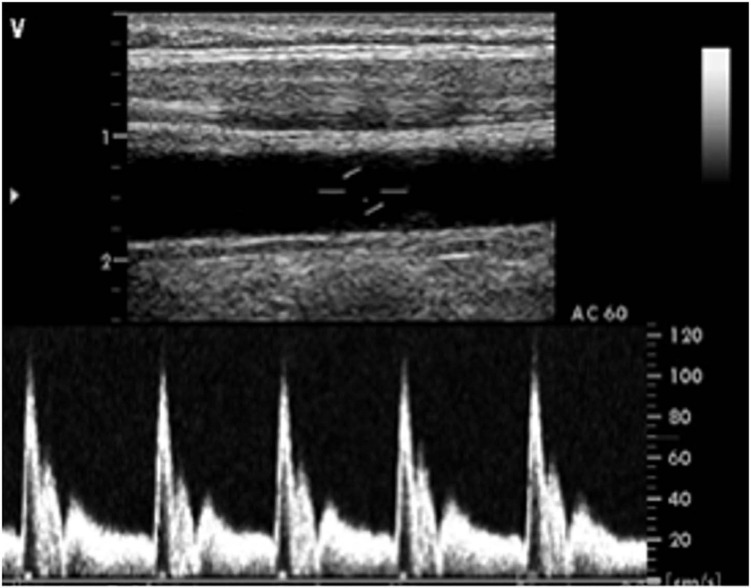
Vascular duplex sonography scan of the left carotid artery showing the longitudinal image of the artery (top) and the corresponding Doppler pulse wave velocity trace (bottom).
